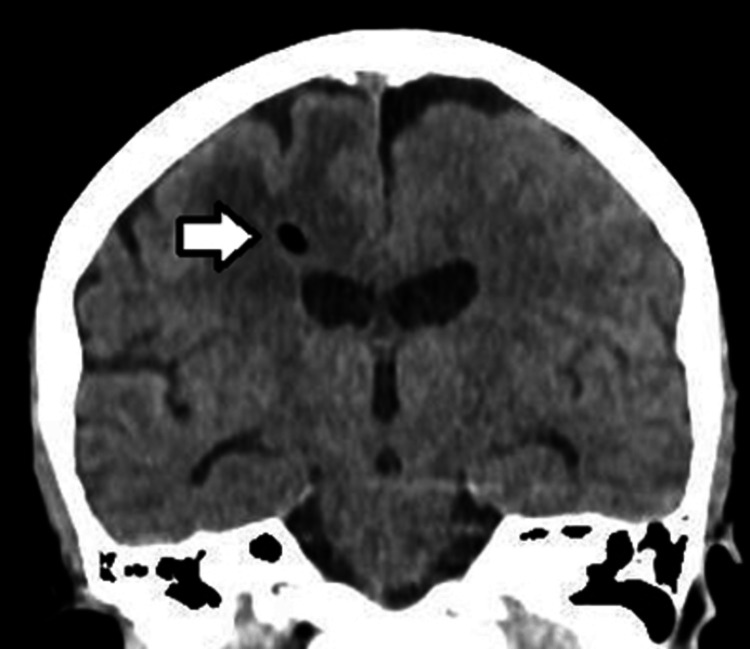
Brain CT scan showing resolution of the brain abscess and the hydrocephalus, with a small area of residual pneumoenchepalon (arrow)
